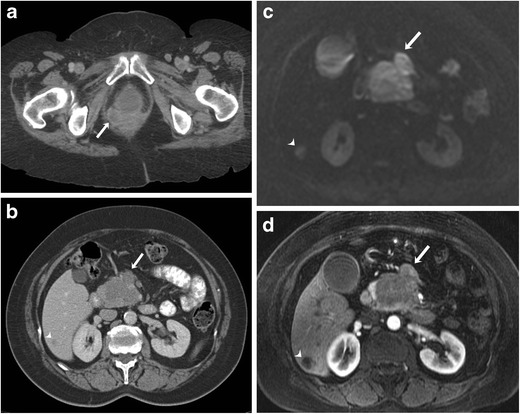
A 63-year-old female with vaginal melanoma and pancreatic and hepatic metastases. a Axial contrast-enhanced CT image shows heterogeneously enhancing vaginal mass (arrow). b Axial contrast-enhanced CT image shows a heterogeneous mass involving the pancreatic head (arrow) and an ill-defined hypointense lesion in the right lobe of the liver (arrowhead). c On corresponding axial diffusion-weighted MRI, the lesions show restricted diffusion. d Axial contrast-enhanced fat-suppressed T1-weighted MR image shows pancreatic (arrow) and hepatic (arrowhead) metastatic lesions
